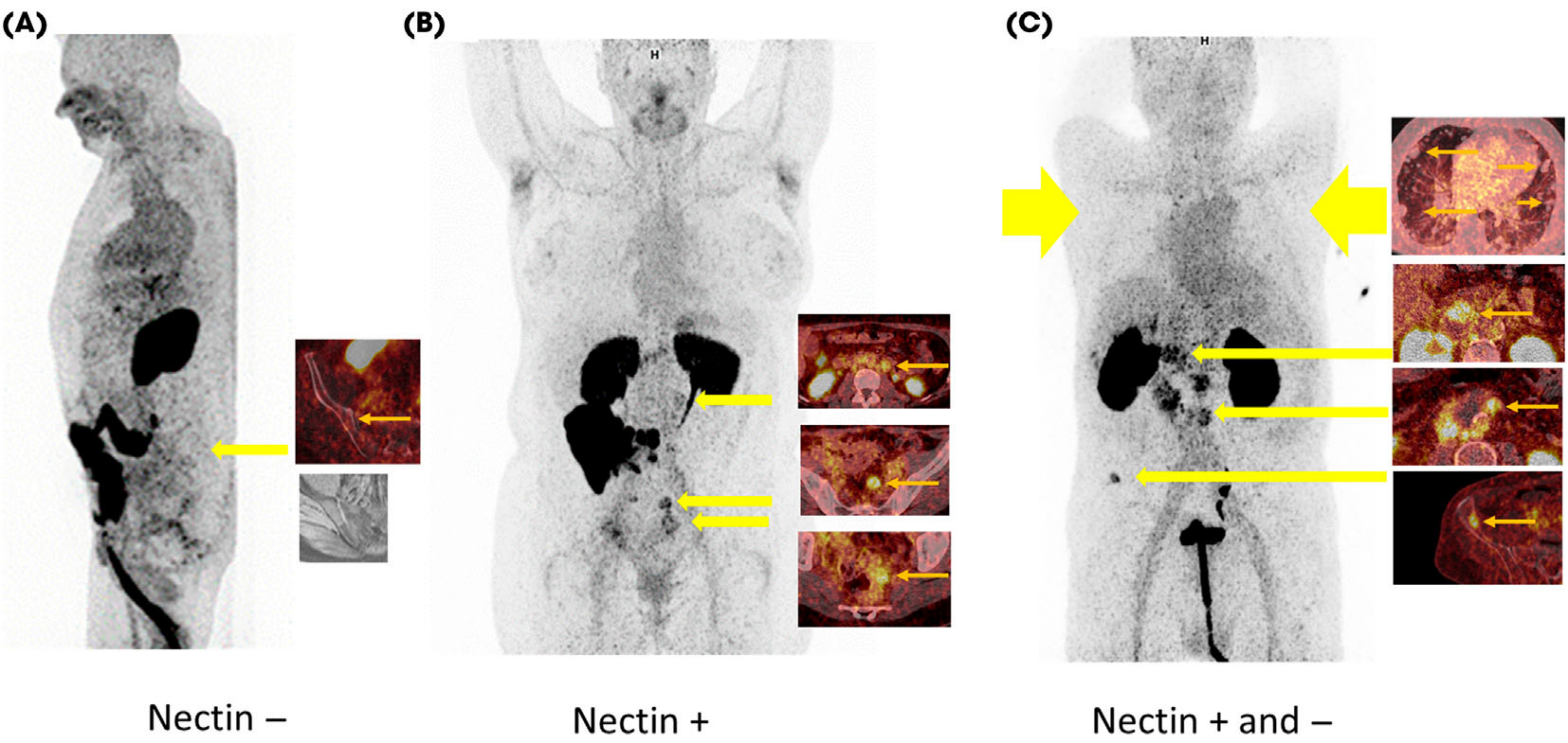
Example images depicting maximum intensity overviews and fusion images of representative metastases (standardised uptake value range 0–5). (A) Unremarkable Nectin‐4 PET/CT with known bone metastases, for example, at the right iliac bone. (B) Nectin‐4‐positive retroperitoneal lymph node metastases. (C) Nectin‐4‐positive lymph node metastases, one Nectin‐4‐positive bone metastasis, and images of Nectin‐4‐negative pulmonary metastases.
